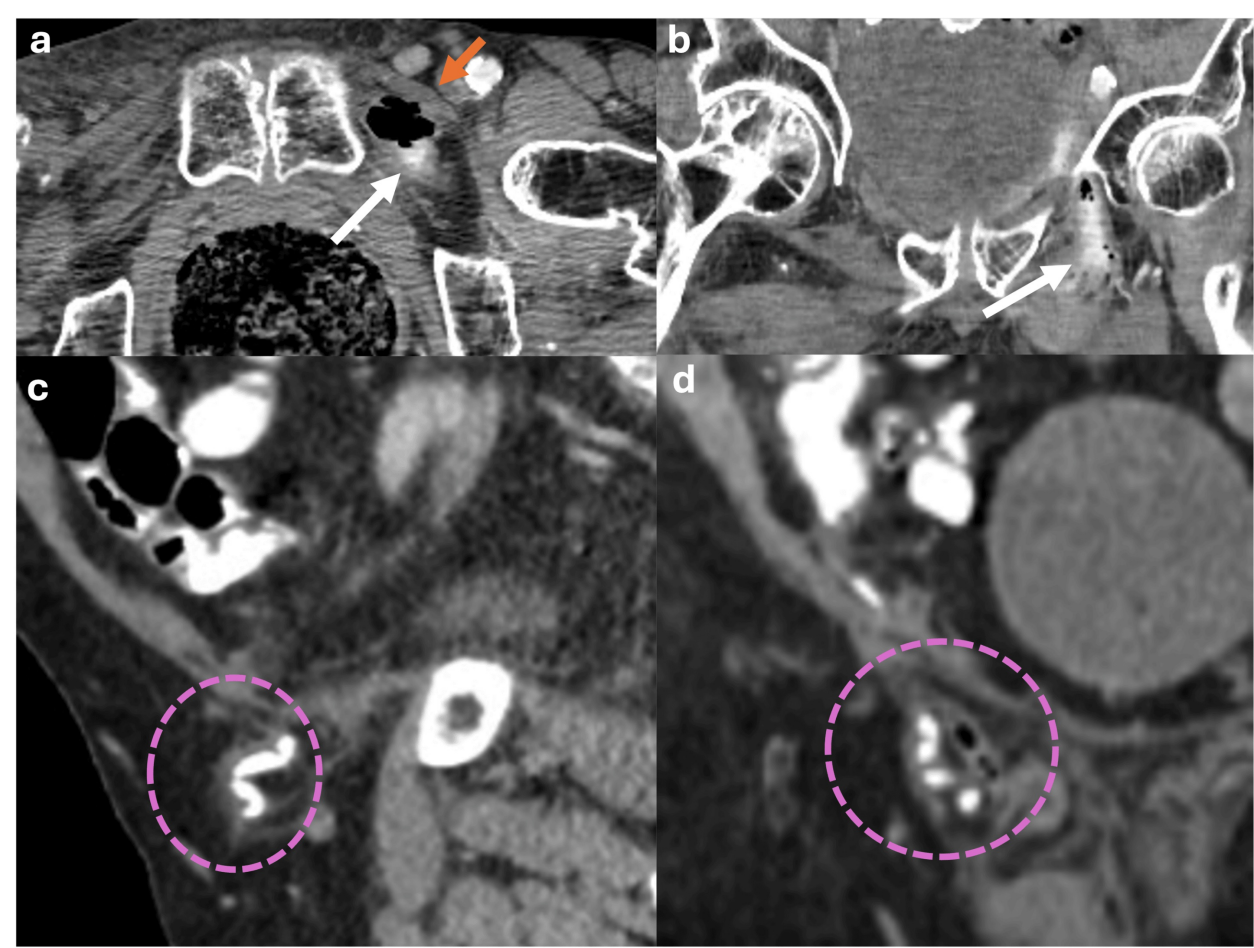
Axial, coronal and sagital abdominal CT scan with oral contrast showing an obturator hernia and Amyand's hernia. (a,b) Axial and coronal CT scan reveals a hernia sac protruding through the obturator foramen (white arrows) located posterior to the pectineus muscle (orange arrow) consistent with an obturator hernia. (c, d) Sagittal and coronal abdominal CT scan from another patient showing a non-inflamed cecal appendix filled with contrast medium, protruding through the inguinal canal consistent with an Amyand's hernia (pink dotted circle). The figure was created by the authors.
